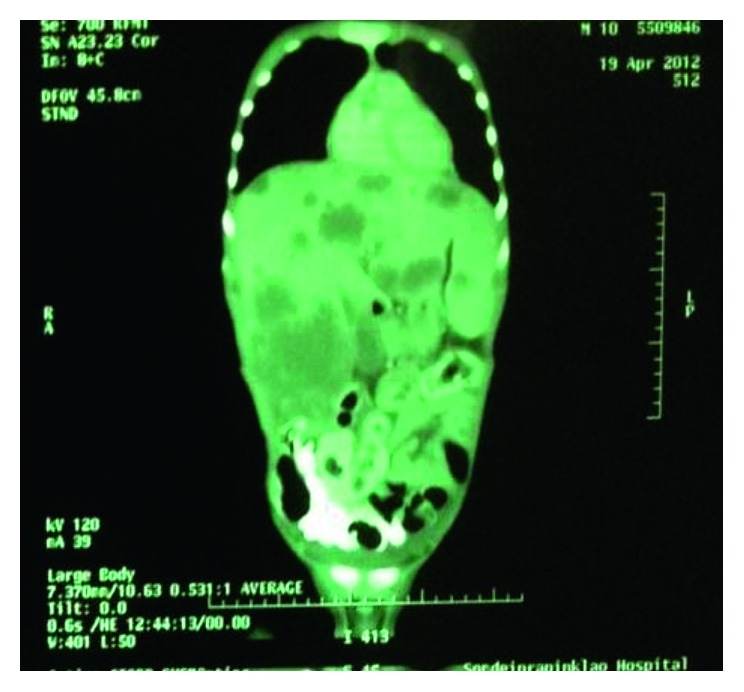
CT chest and whole abdomen (coronal view) showing marked hepatosplenomegaly, enlarged size of the pancreas, and numerous heterogeneous masses at both lobes of the liver and the pancreas.
